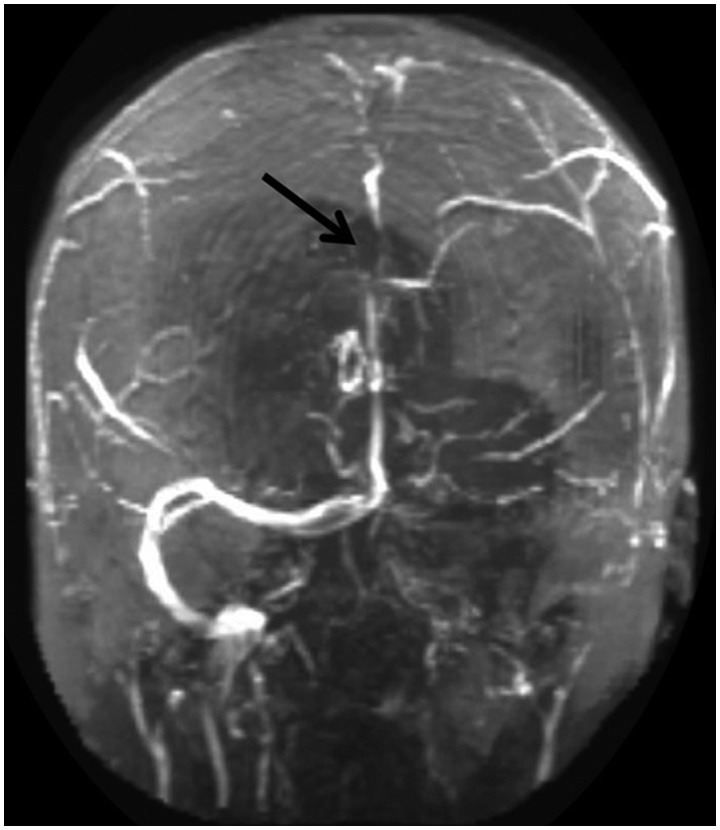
Magnetic resonance imaging with venography on admission, confirming the intraluminal thrombosis of the sagittal (arrow), transverse, and sigmoid sinuses, and extending into the cranial portion of the internal jugular vein.
